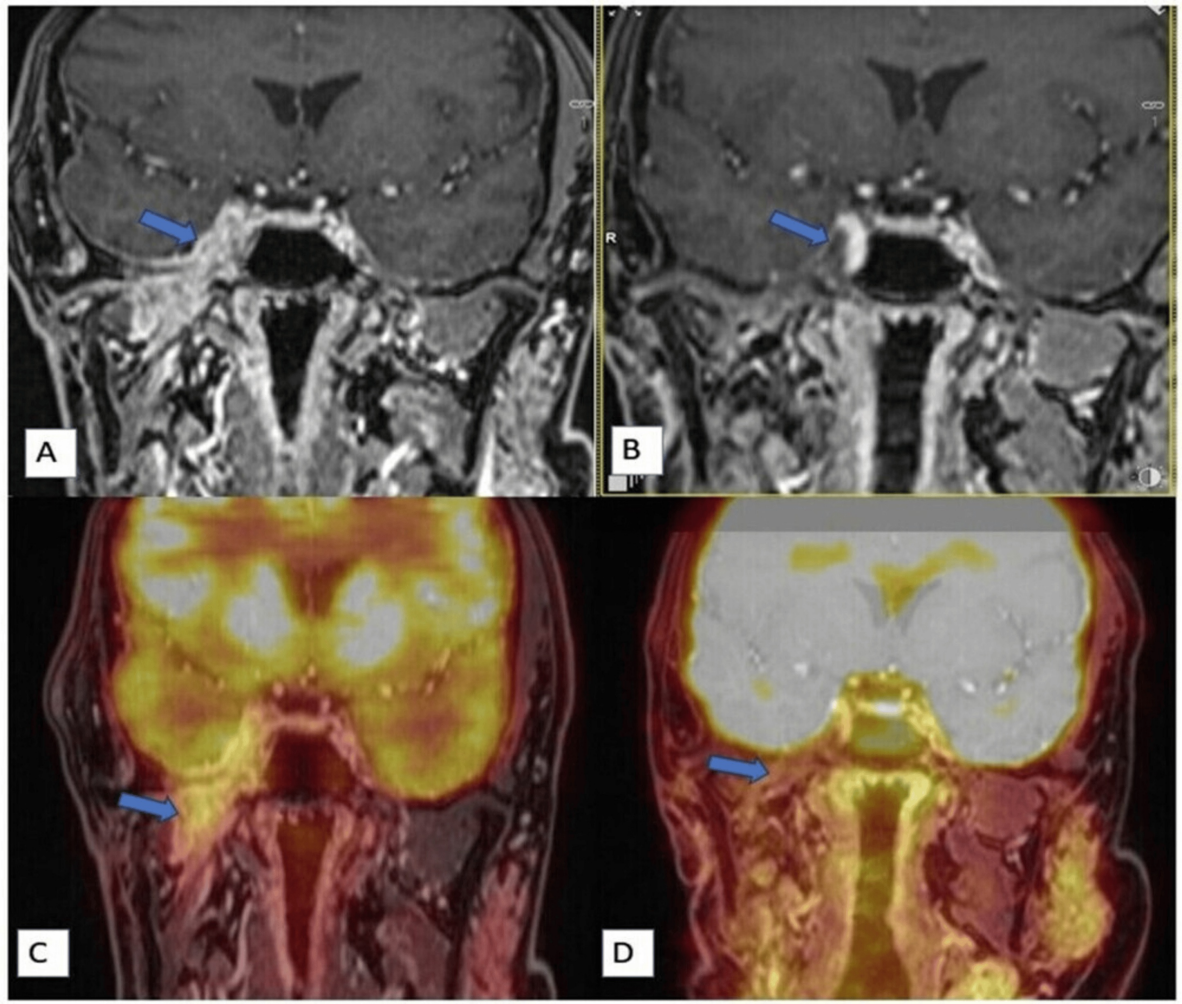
Regression on radiology A, C. (Sept 2023, before the combination regimen started) Post-contrast T1 coronal image (A) and corresponding fused PET-MR image (C) show 18F-fluorodeoxyglucose (FDG)-avid enhancing thickening (arrow) near the skull base with intracranial extension in the right Meckel's cave and cavernous sinus region. B, D. (Aug 2024, with treatment): Post-contrast T1 coronal image (B) and corresponding fused PET-MR image (D) show a complete response.
